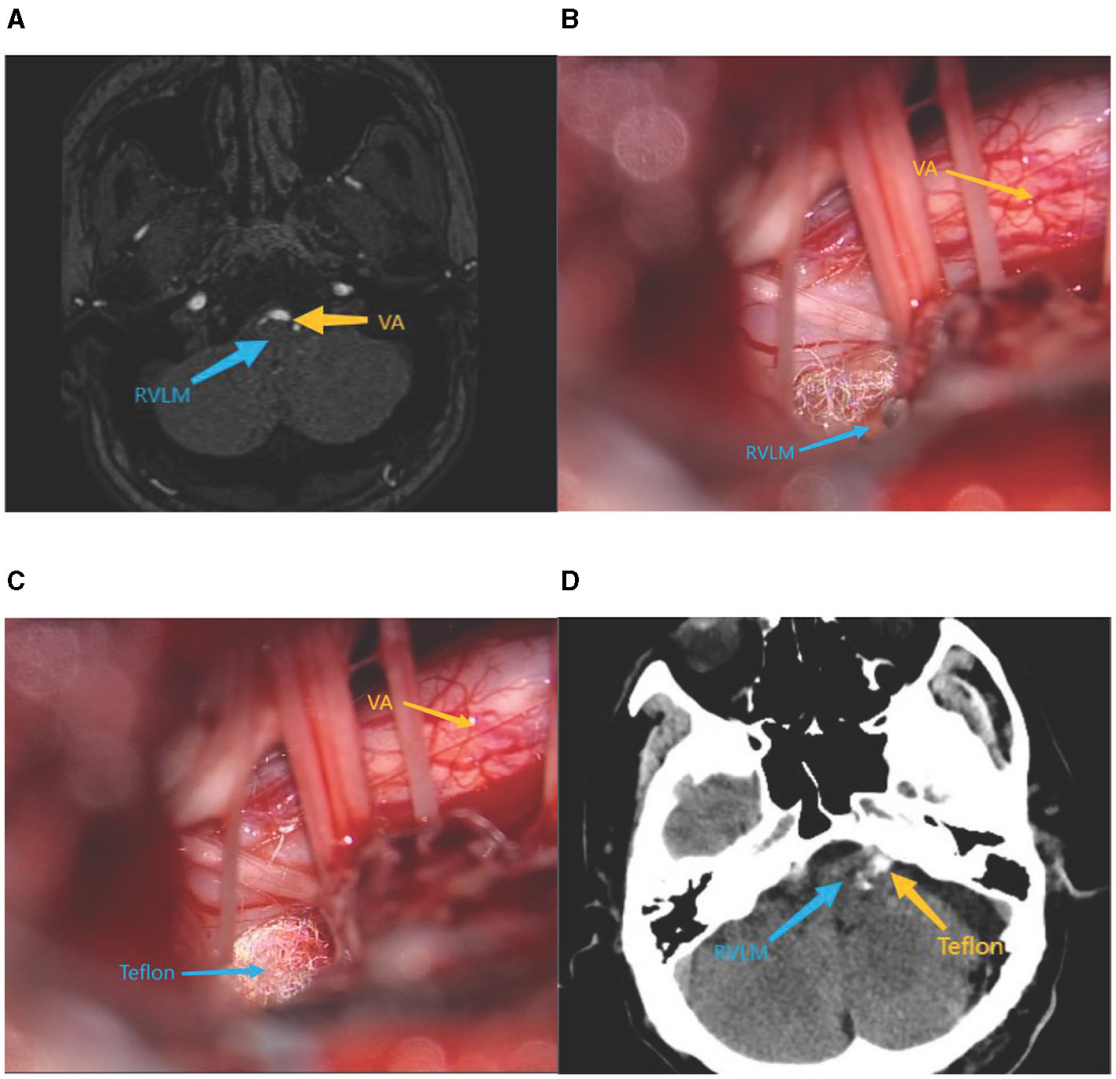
(A) Preoperative cranial MRI. (B) Intraoperative exposure of RVLM. (C) Intraoperative placement of Teflon pads. (D) Postoperative cranial ct.
